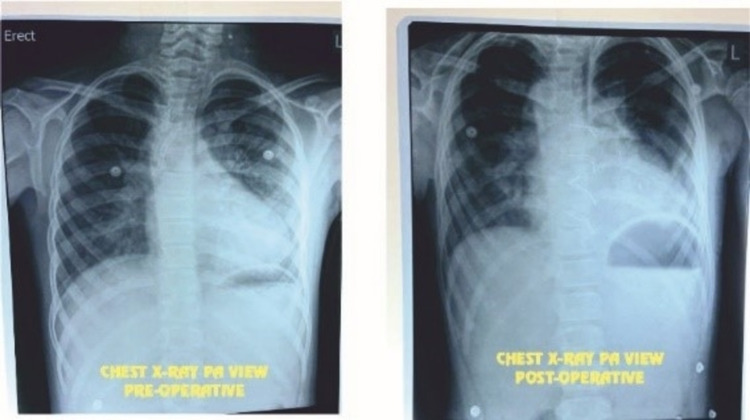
Preoperative and postoperative chest X-rays (PA view).
